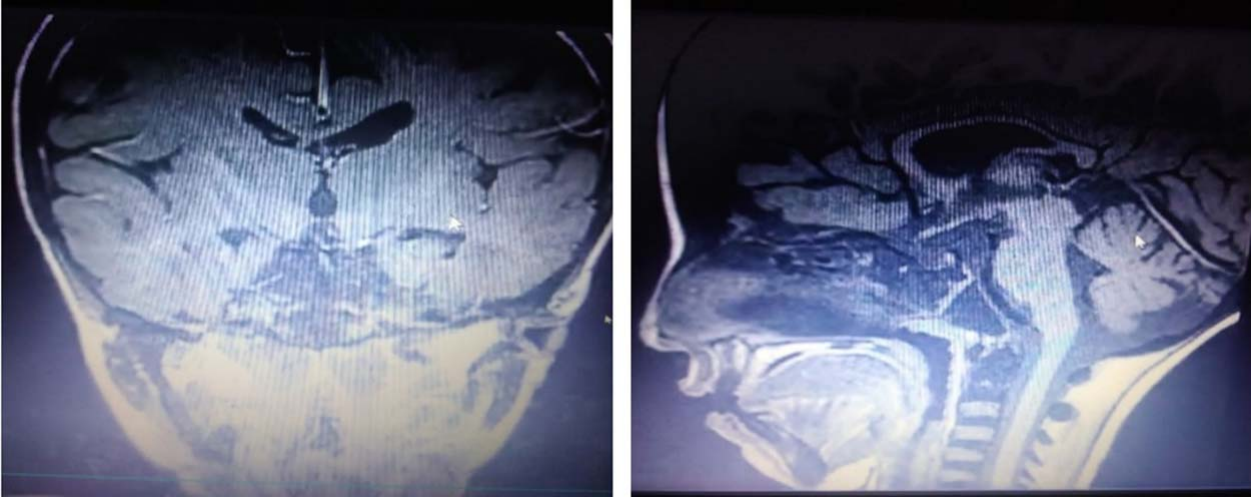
MRI Of the Pituitary gland with IV contrast showed severe atrophy of the pituitary gland.
